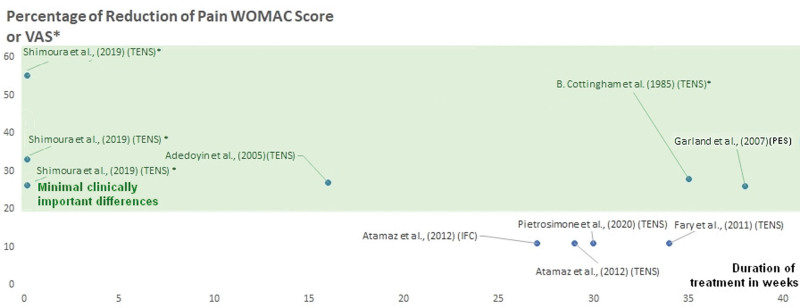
Comparative analysis of diathermy (heat), electrical stimulation, and ultrasound modalities in knee and hip osteoarthritis. Box plots with standard error bars depict mean and standard deviation for each modality, revealing no statistically significant differences. Studies surpassing the minimum clinical efficiency threshold (set at 20%, based on literature evidence) are emphasized within a highlighted frame, facilitating identification and comparison of efficacious interventions.
